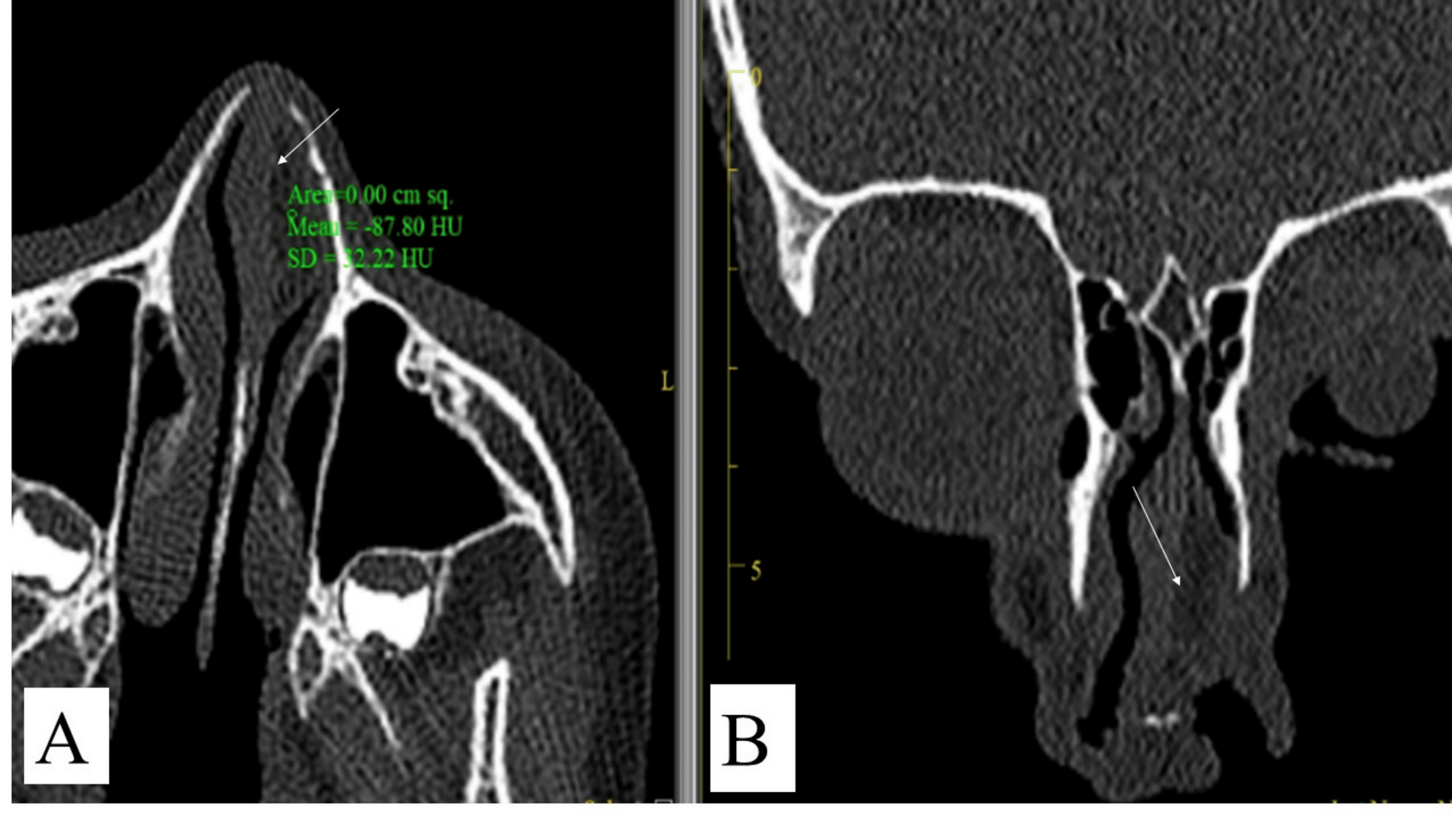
CT of nasal septal lipoma A) axial section; B) coronal view showing left anterior septal fatty lesion with mean Hounsfield unit (HU) – 88, homogenously hypodense representing lipomatous lesion
